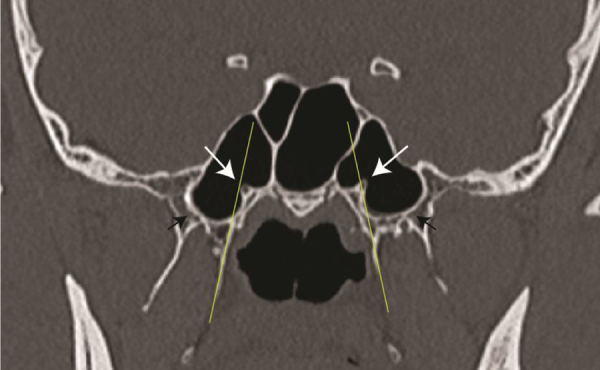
Coronal CT scan showing the position of the vidian canal (white arrow) in the same plane as that of the medial pterygoid plate (thin yellow line) on the left side and medial to this plane on the right side. Black arrows indicated a pneumatised pterygoid plate.
